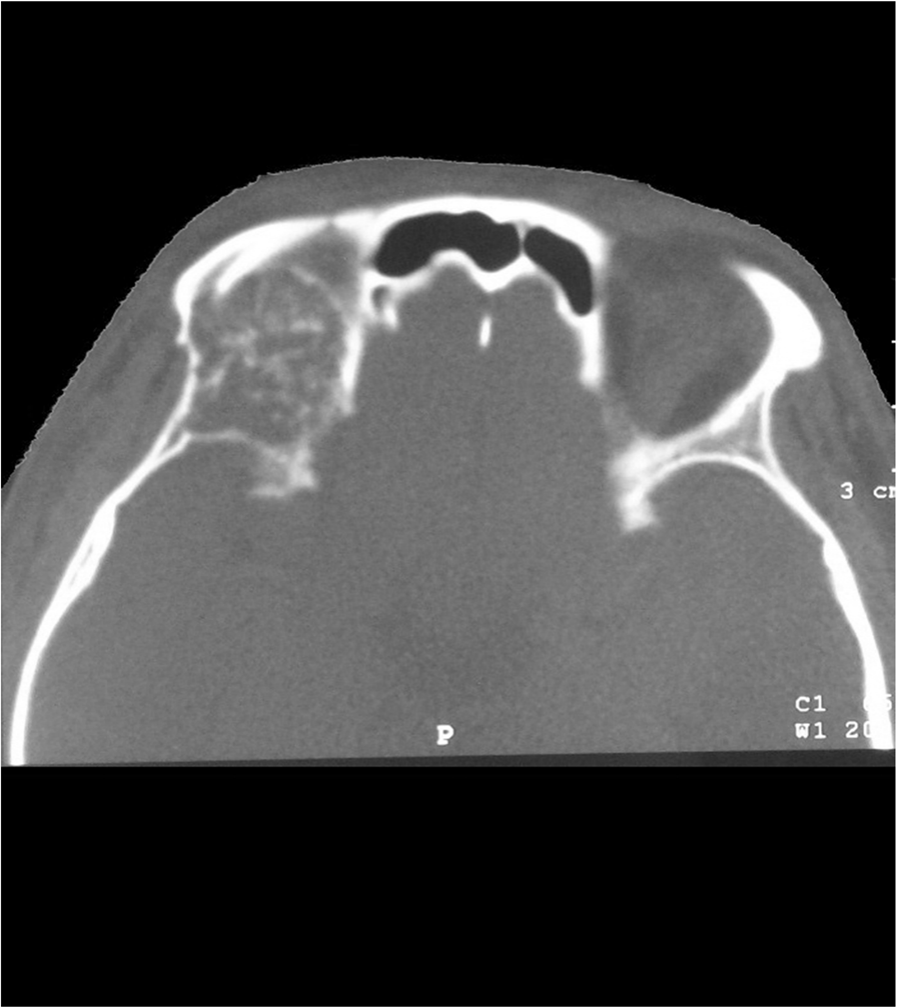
Bone window computed tomography scan demonstrating a 3 cm mass destroying the surrounding bone and penetrating into the internal skull in the upward wall of the right orbit.
